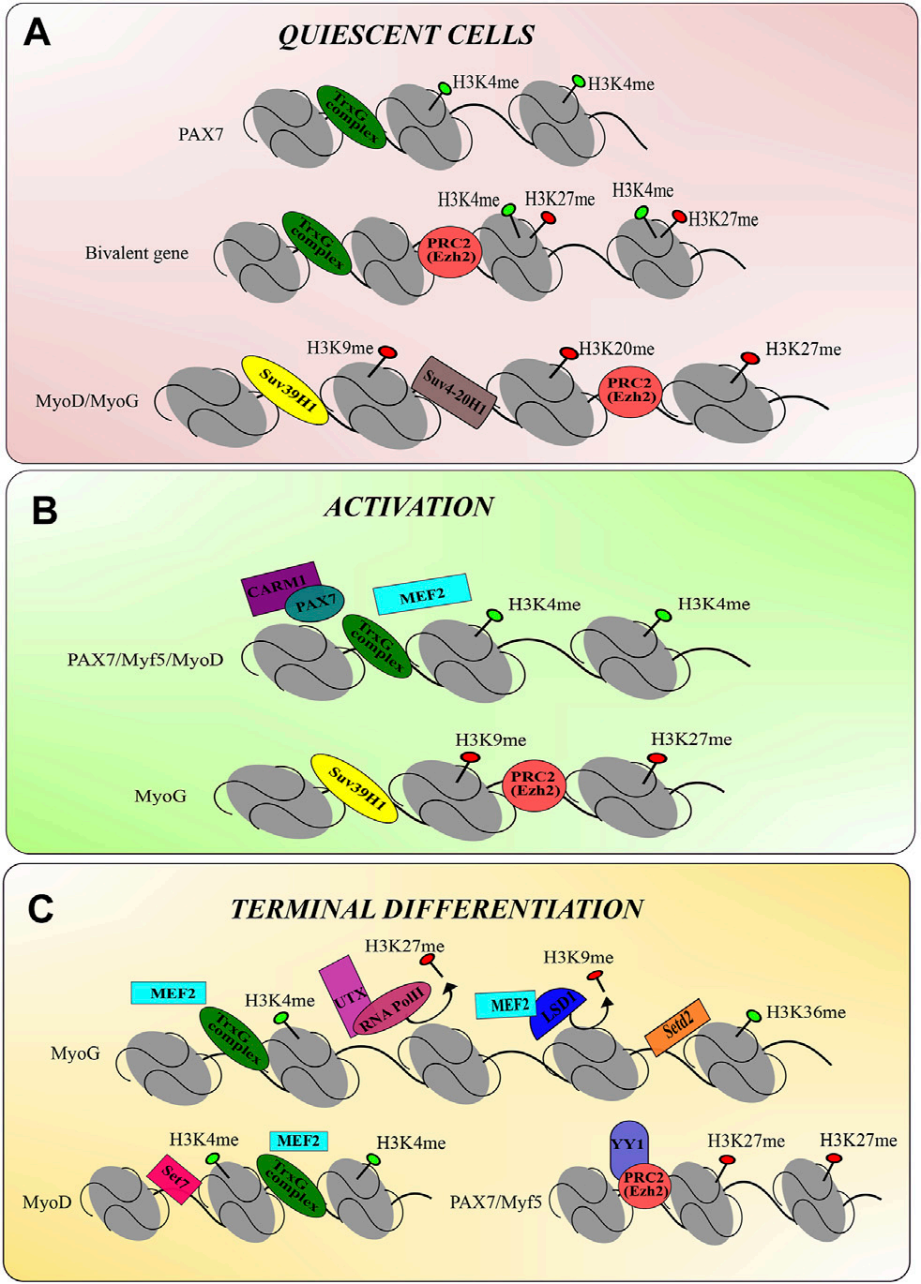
The epigenetic modulation of histone methyltransferases during skeletal muscle regeneration. (A) Epigenetic mechanisms of histone methyltransferases in quiescent stem cells. TrxG-mediated H3K4me catalyses the permissive chromatin of Pax7. MyoD and MyoG gene expression is repressed by H3K27me, H3K9me, and H3K20me via their respective enzymes. Interestingly, the bivalent gene consists of an activating H3K4me mark and the repressive H3K27me, however, H3K27me activity dominates to repress gene transcription during the quiescent state. (B) Epigenetic mechanisms of histone methyltransferases in activating progenitor cells. Upon the activation of stem cells, the permissive chromatin of Pax7, Myf5 and MyoD is catalysed by deposition of H3K4me via TrxG complexes. MEF2 and Carm-Pax7 complex recruits TrxG complexes to establish the permissive chromatin. MyoG remains with the repressive chromatin marks, H3K27me and H3K9me, catalysed by PRC2 and SUV39H1, respectively. (C) Epigenetic mechanisms of histone methyltransferases in during terminal differentiation. The MyoG chromatin permits gene transcription via H3K4me and H3K36me marks by TrxG complexes and Setd2, respectively. UTX and RNA PolII remove the repressive mark H3K27me, and MEF2 recruits LSD1 to remove the H3K9me repressive mark. MyoD gene transcription continues via the activating H3K4me. Pax7 and Myf5 are silenced by recruitment of PRC2 by YY1, which deposits the H3K27me repressive mark.
